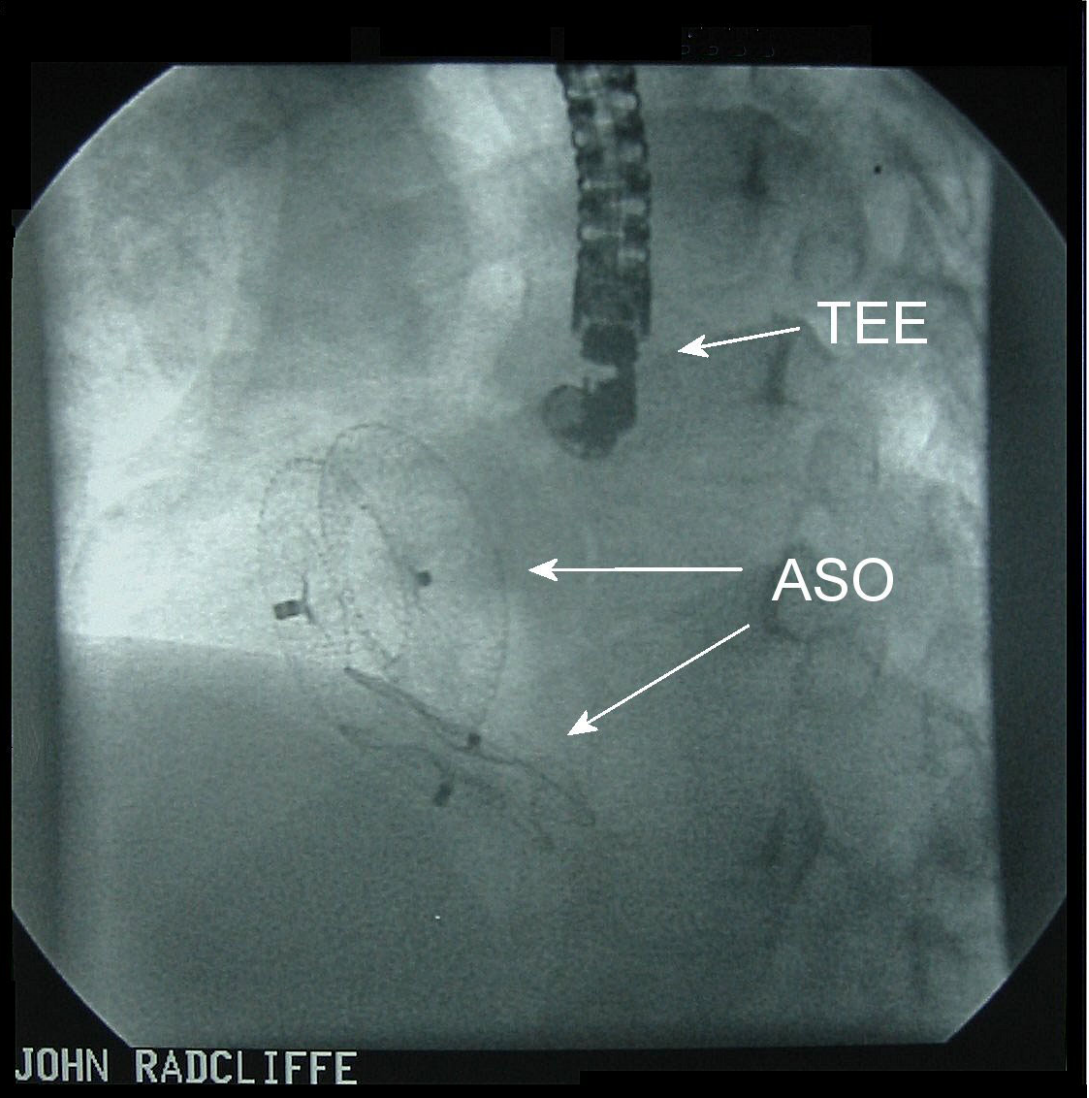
Stored fluoroscopy following placement of the two Amplatzer septal occluders (ASOs). TEE – Transesophageal echocardiography probe.
